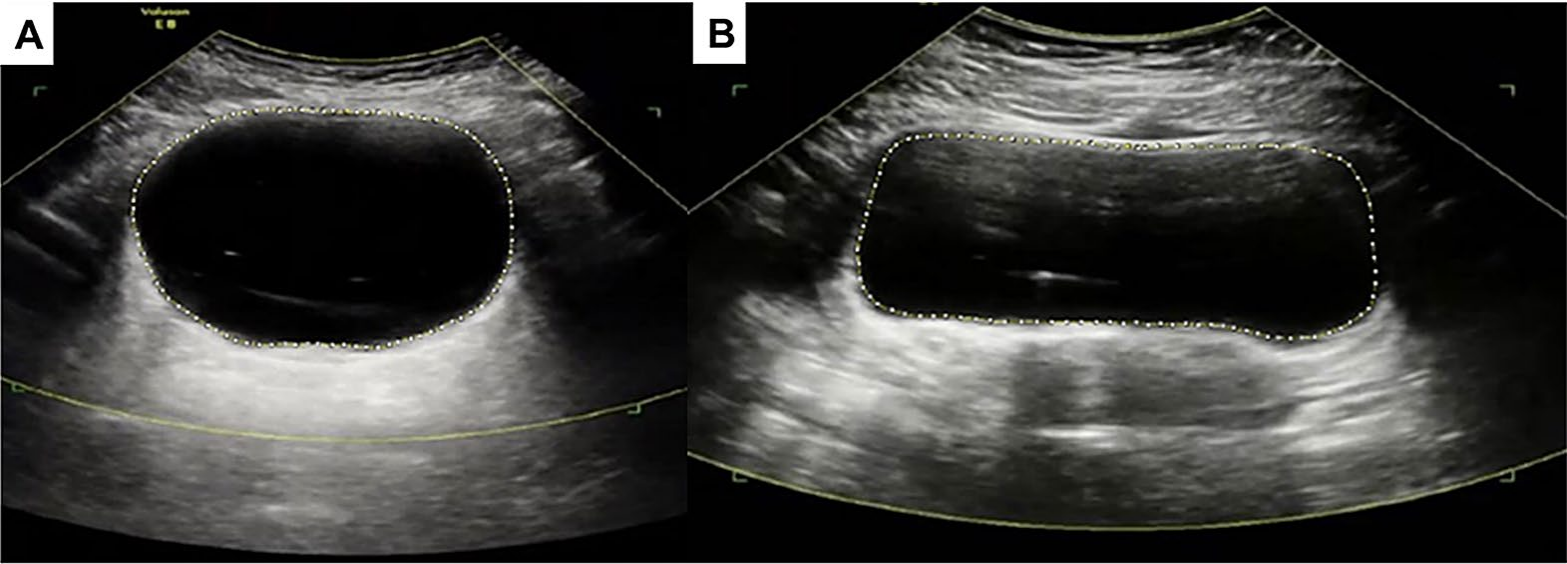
Ultrasound images with the bladder wall traced in ImageJ for (A) an individual with detrusor overactivity and circularity of 0.923 and (B) an individual without detrusor overactivity and circularity of 0.817
